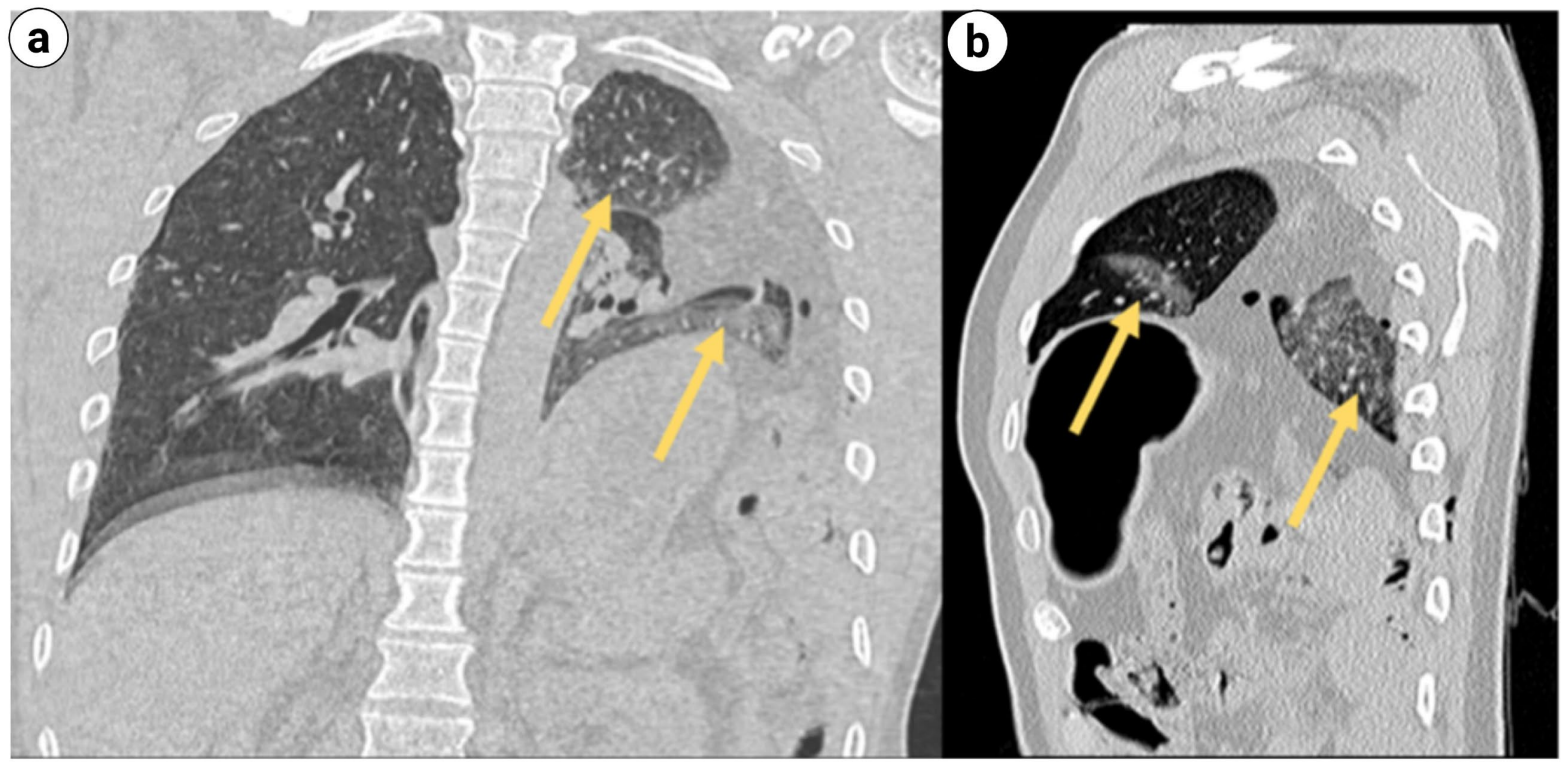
Multilobar contusion of the left lung (indicated by the yellow arrow): (a) chest CT coronal plane; (b) chest CT sagittal plane.
